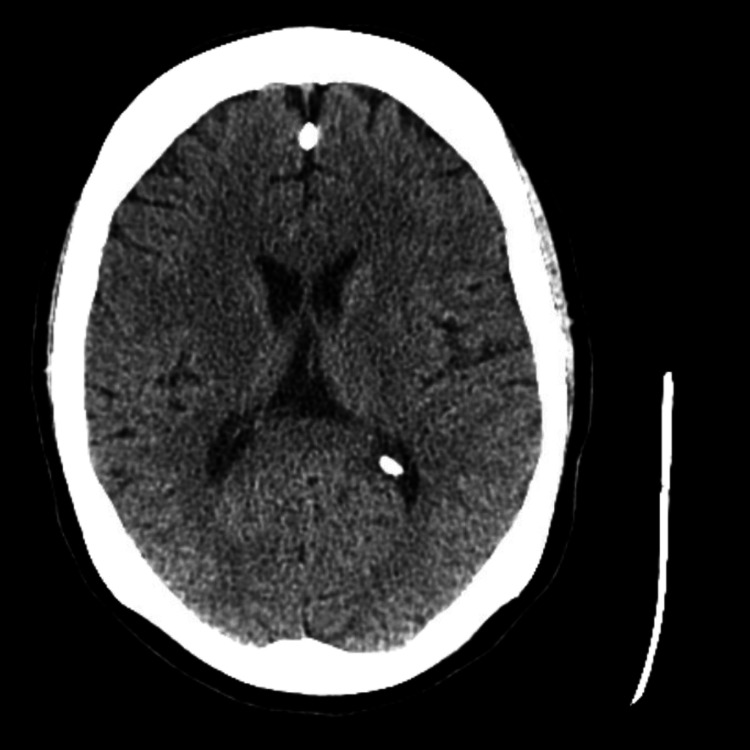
CT brain without contrast: no acute findings in the head/brain.
